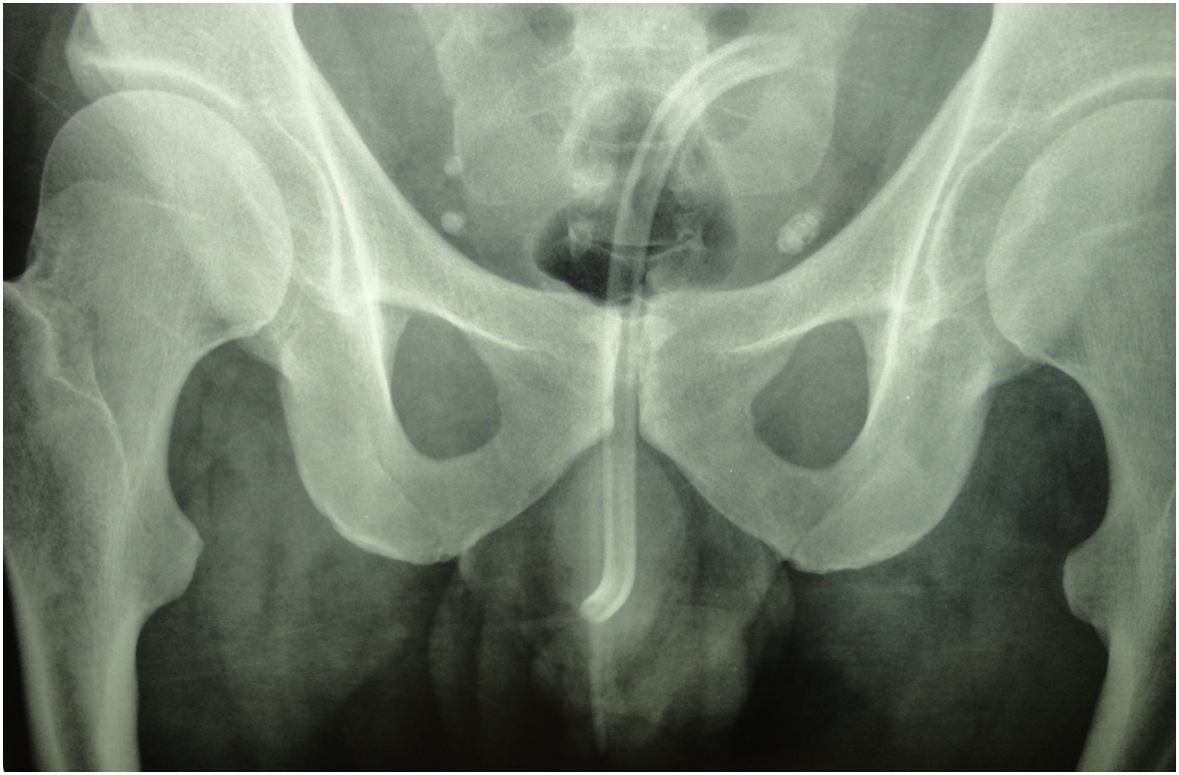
Plain film of the urinary tract showing a hollow tubular object corresponding to a cable in the urethra.
